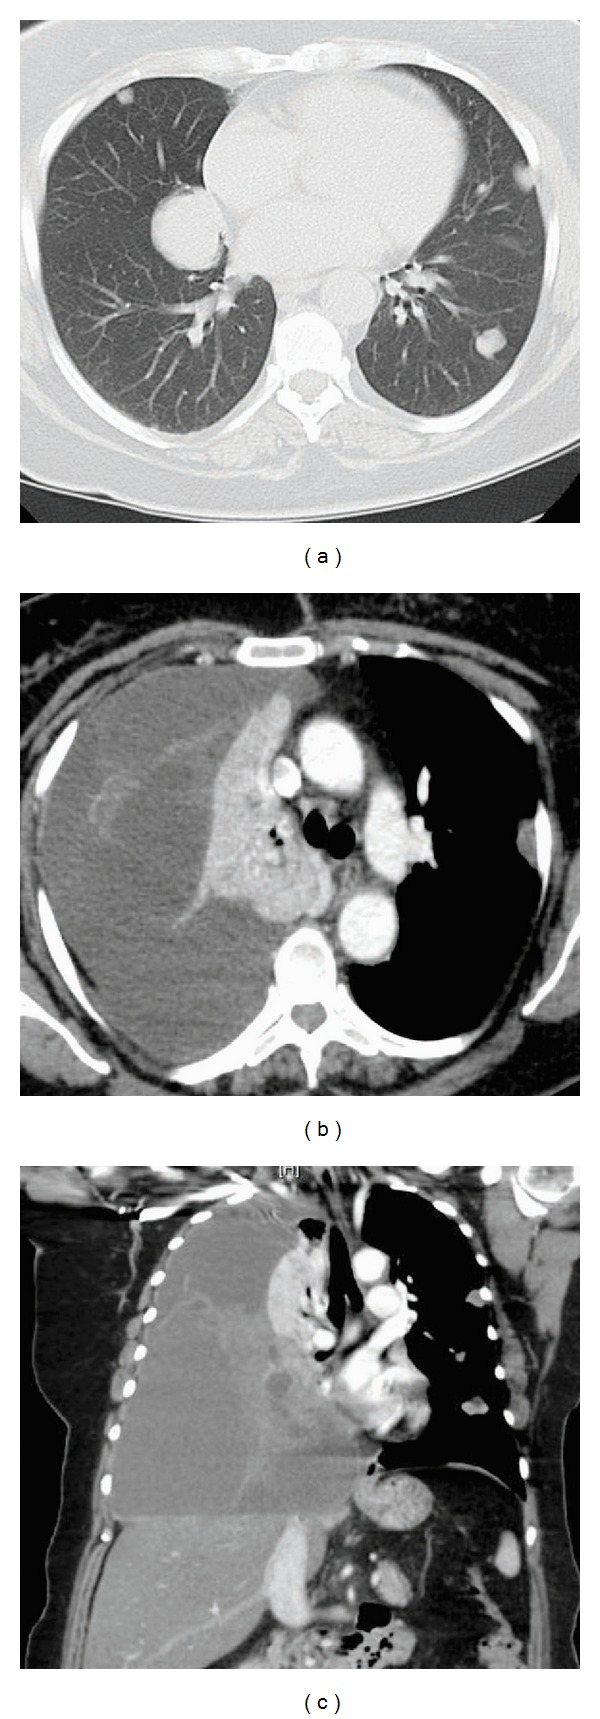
(a) Transverse section of thorax by CT before presentation. (b) Transverse section of thorax by CT at presentation showing fluid-filled right hemithorax with an irregular mass. (c) Coronal section of thorax by CT at presentation showing fluid-filled right hemithorax with an irregular mass.
